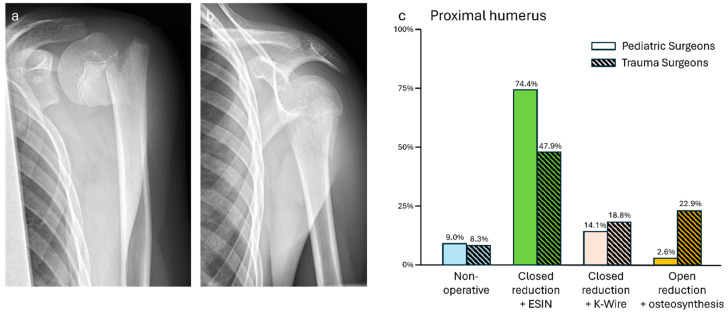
(a) Anterior–posterior and (b) lateral X-rays of a proximal subcapital humeral fracture (f, 13 y, 150 cm, 48 kg). (c) Descriptive data of the survey results, indicated in percentages.
